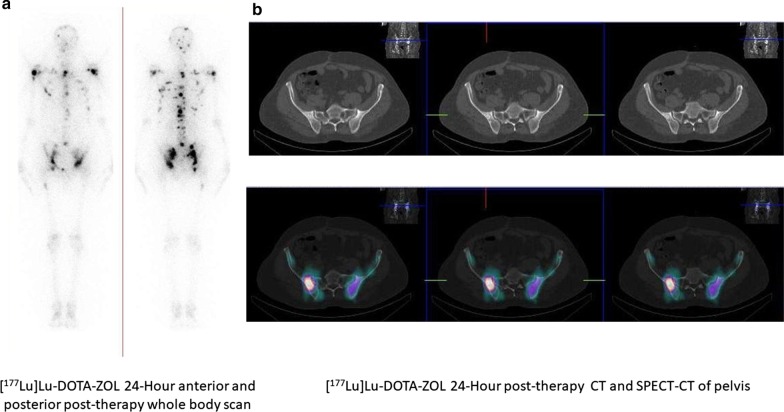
A 55-year-old male diagnosed with prostatic adenocarcinoma underwent radical prostatectomy in April 2017, and his Gleason score was 3 + 4 = 7. He underwent hormonal therapy until April 2018 and received 1# of radiotherapy to B/L hip and D5-vertebra. He was referred to us for symptomatic pain relief. His baseline pain scoring (VASmax) was 9/10, with maximum pain in the B/L hips. We administered 1.3 GBq of [^177^Lu]Lu-DOTA-ZOL and 24-h post-administration, a the 24-h anterior and posterior post-therapy whole-body scans demonstrating avid [^177^Lu]Lu-DOTA-ZOL uptake in multiple skeletal sites. b The uptake of [^177^Lu]Lu-DOTA-ZOL in the pelvic bone metastases on post-therapy SPECT/CT
